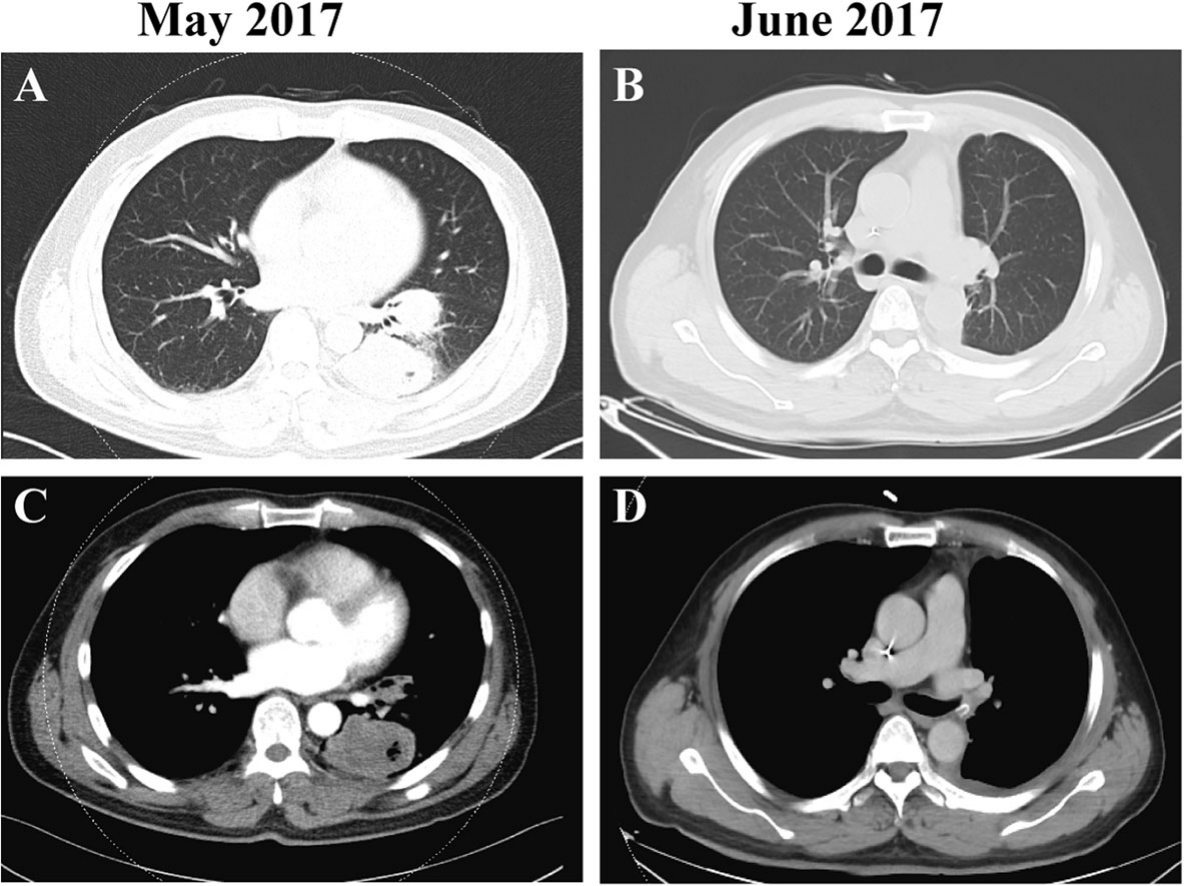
CT scans of the patient. a, c CT scan before lobectomy. Two masses in the left lower lung lobe were noted; and b, d CT scan after lobectomy
